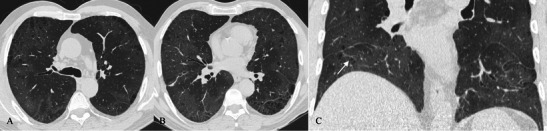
DIP pattern in a 60-year-old man. The patient (smoker of 25 pack-years) complained of progressive dyspnoea and dry cough. Pulmonary function was characterised by a moderate reduction in DLCO and normal dynamic lung volumes. Physical examination revealed digital clubbing and fine crackles were heard in the lung bases on chest auscultation. On HRCT images, lung ground-glass opacifications are clearly shown through the lungs and correlate with the intra-alveolar accumulation of macrophages. Bronchial wall thickening and bronchiectasis are also associated. Microcysts have been described in almost 50 % of cases [7]. The coronal HRCT image (C) clearly shows peribronchovascular small cysts (white arrow); also emphysema is recognisable through the lung
